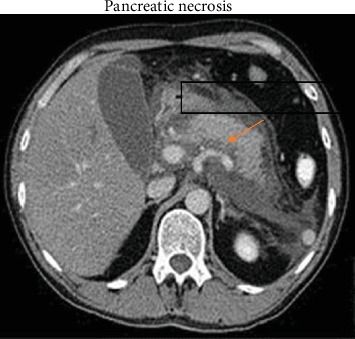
CT of the abdomen showing pancreatic necrosis (red arrow).
